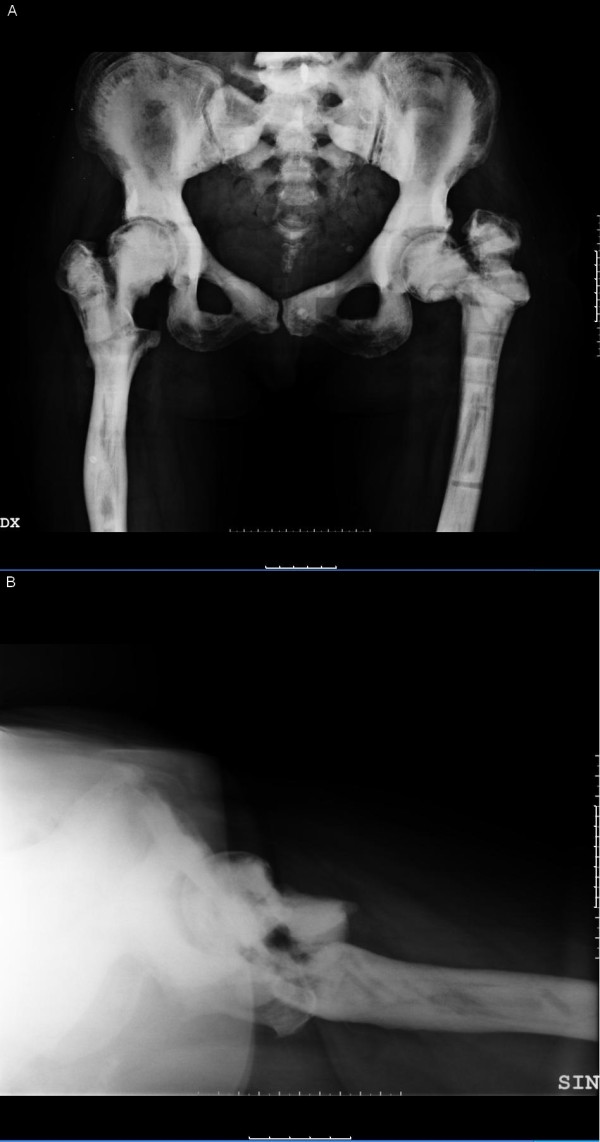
Antero-posterior (A) and axial (B) x-ray views, pre-operatively, after previous failed plate osteosynthesis and external fixator. Septic non-union of the femoral neck and septic hip arthritis.
